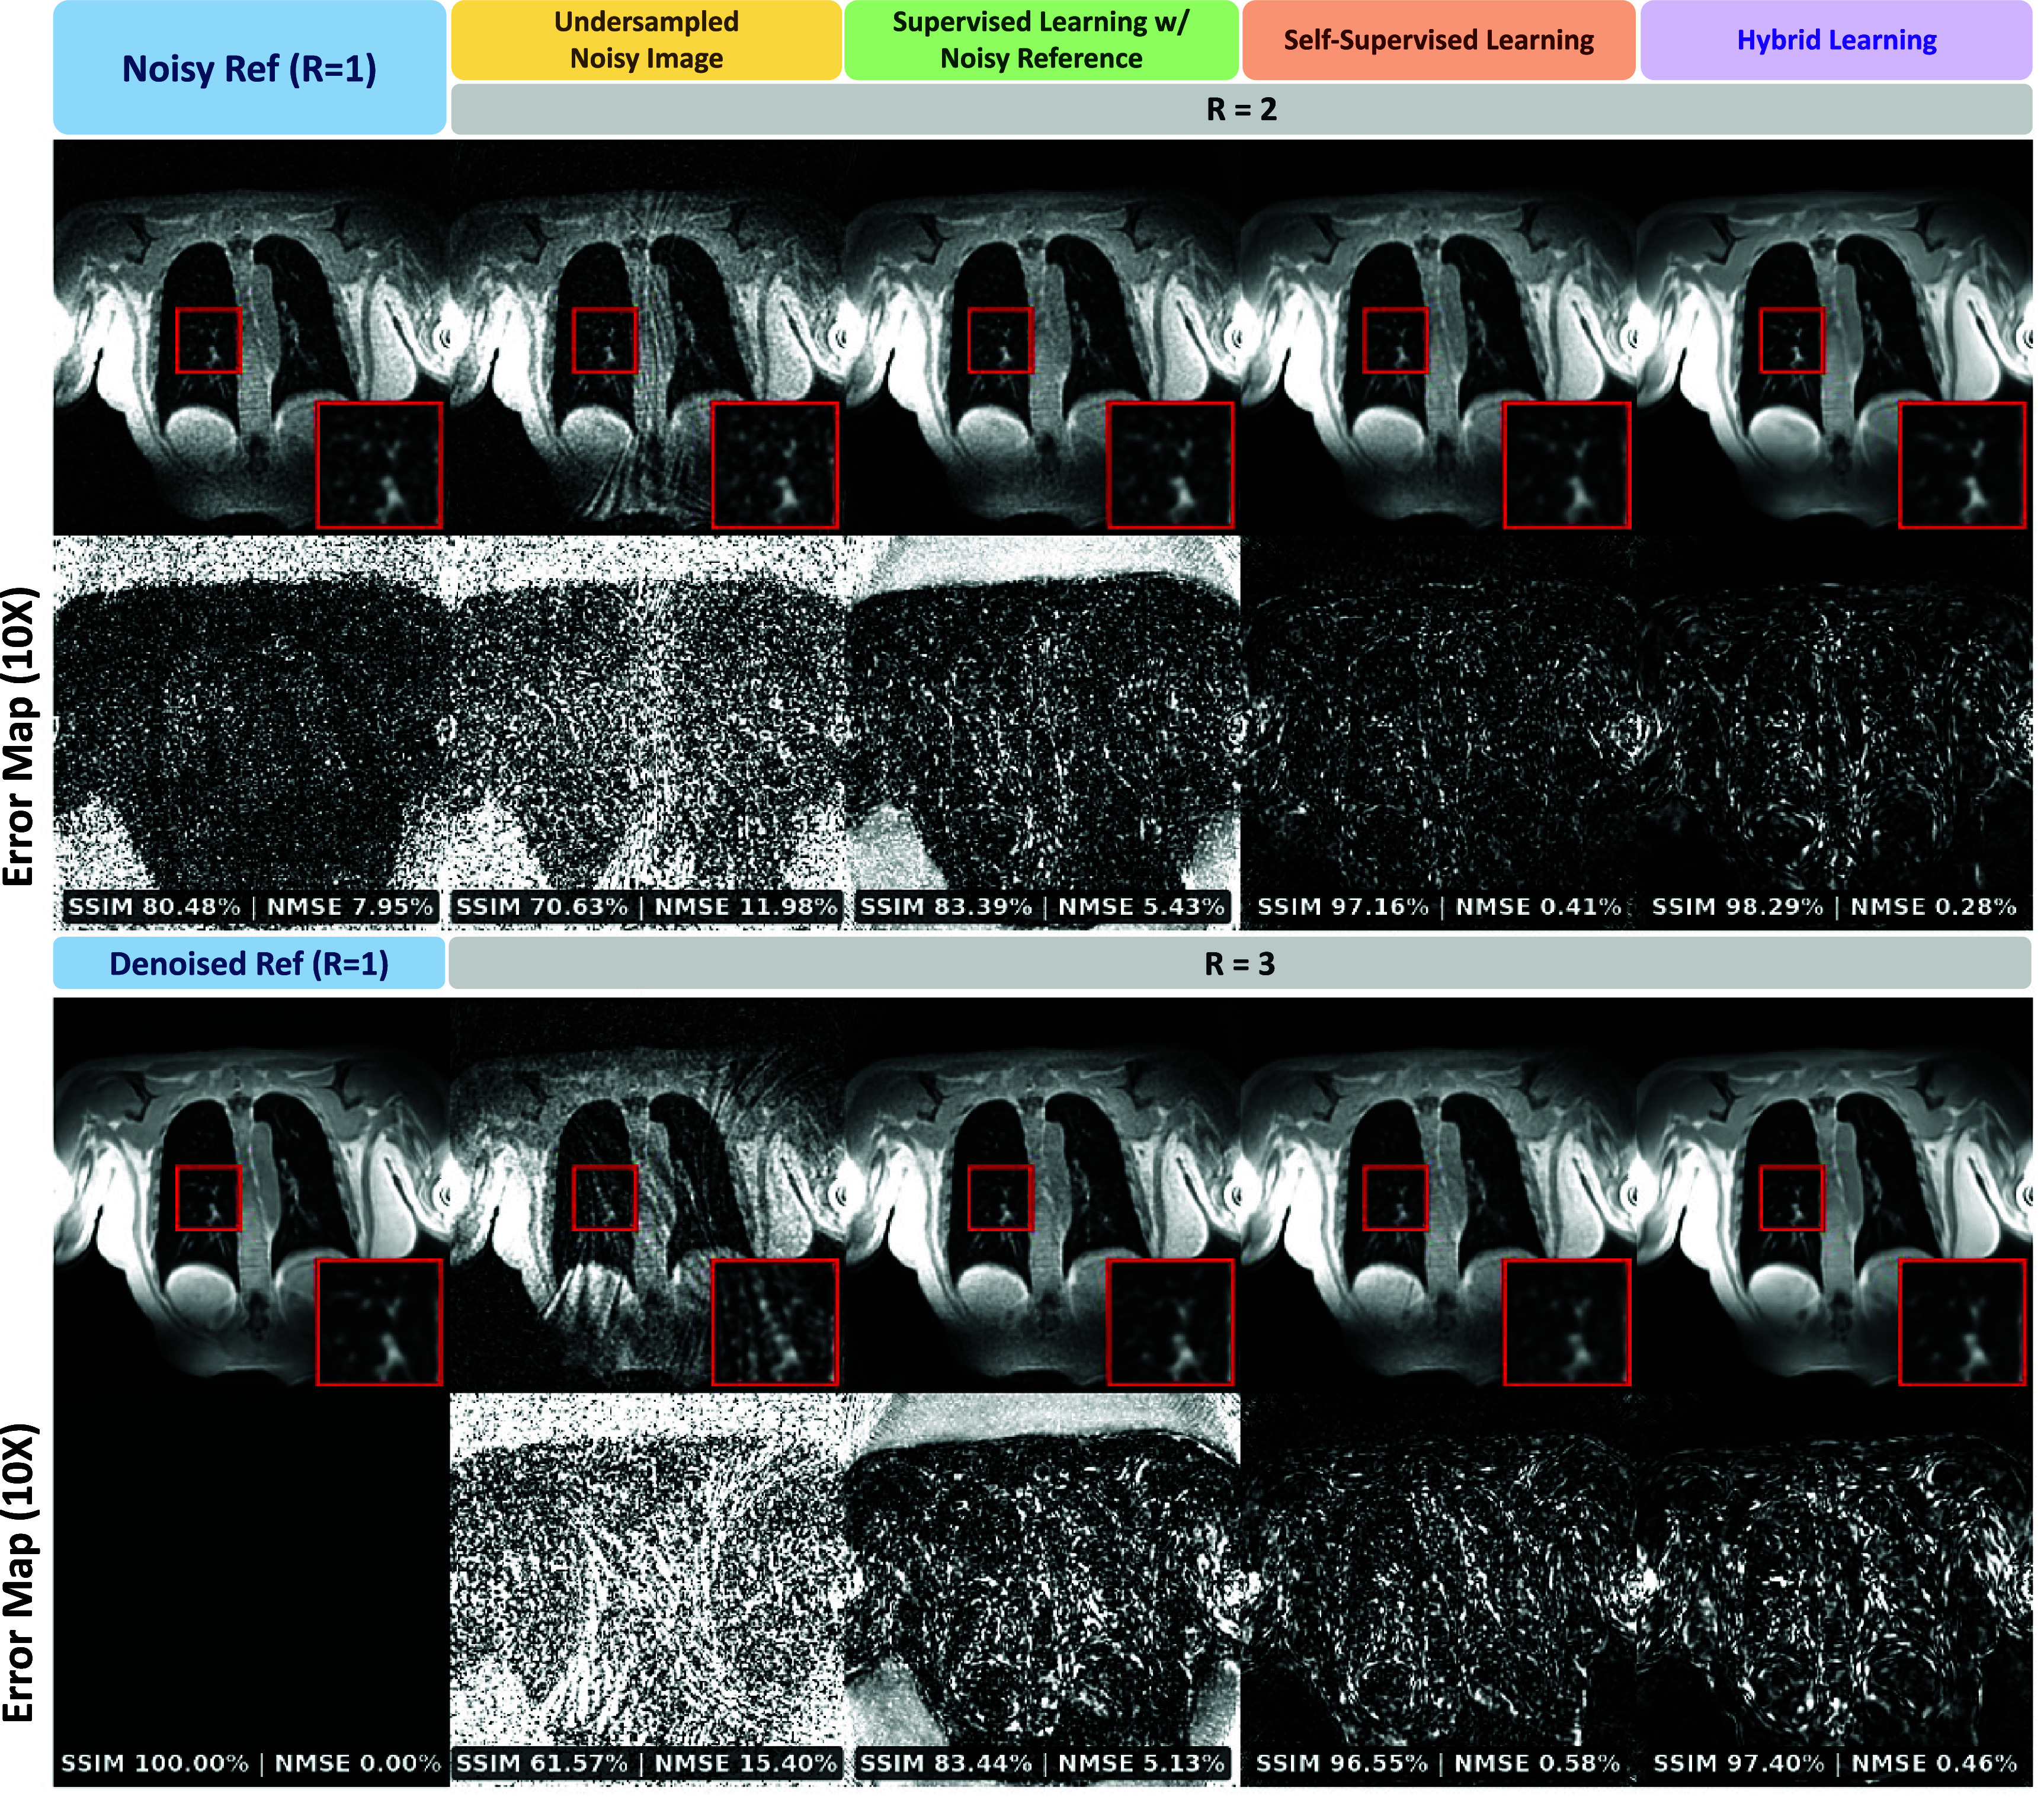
Lung MRI (0.55 T), spiral sampling at *R* = 2 and 3. A representative case from 0.55 T spiral lung MRI at *R* = 2 and *R* = 3, comparing hybrid learning with supervised and self-supervised learning. Regions of interest (ROIs) and 10× difference maps are provided for reference. The fully sampled image (Noisy Reference) shows visible noise, whereas the denoised pseudo-reference image (Denoised Reference) provides improved visual quality. Hybrid learning outperforms both supervised and self-supervised learning at both acceleration rates.
